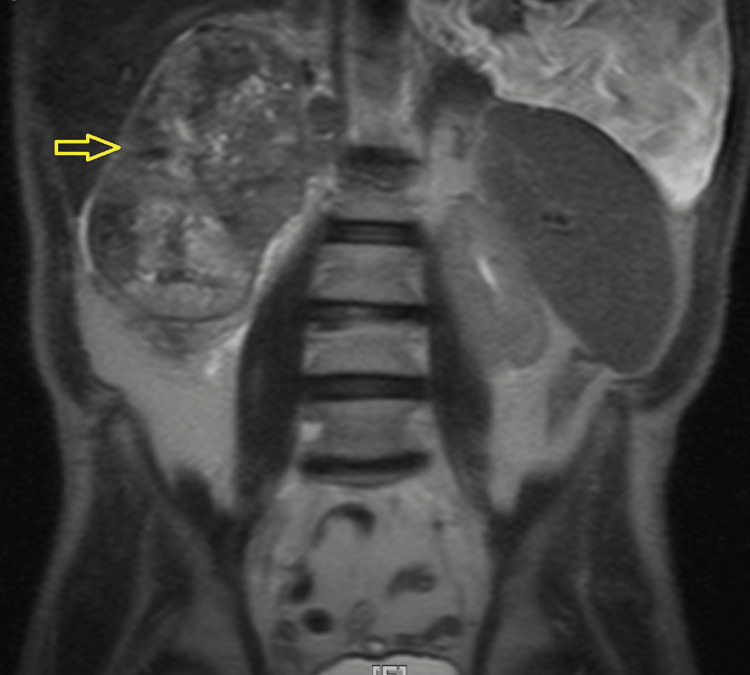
Abdominal MRI demonstrating very large right renal mass encompassing most of the right kidney, concerning for RCC
